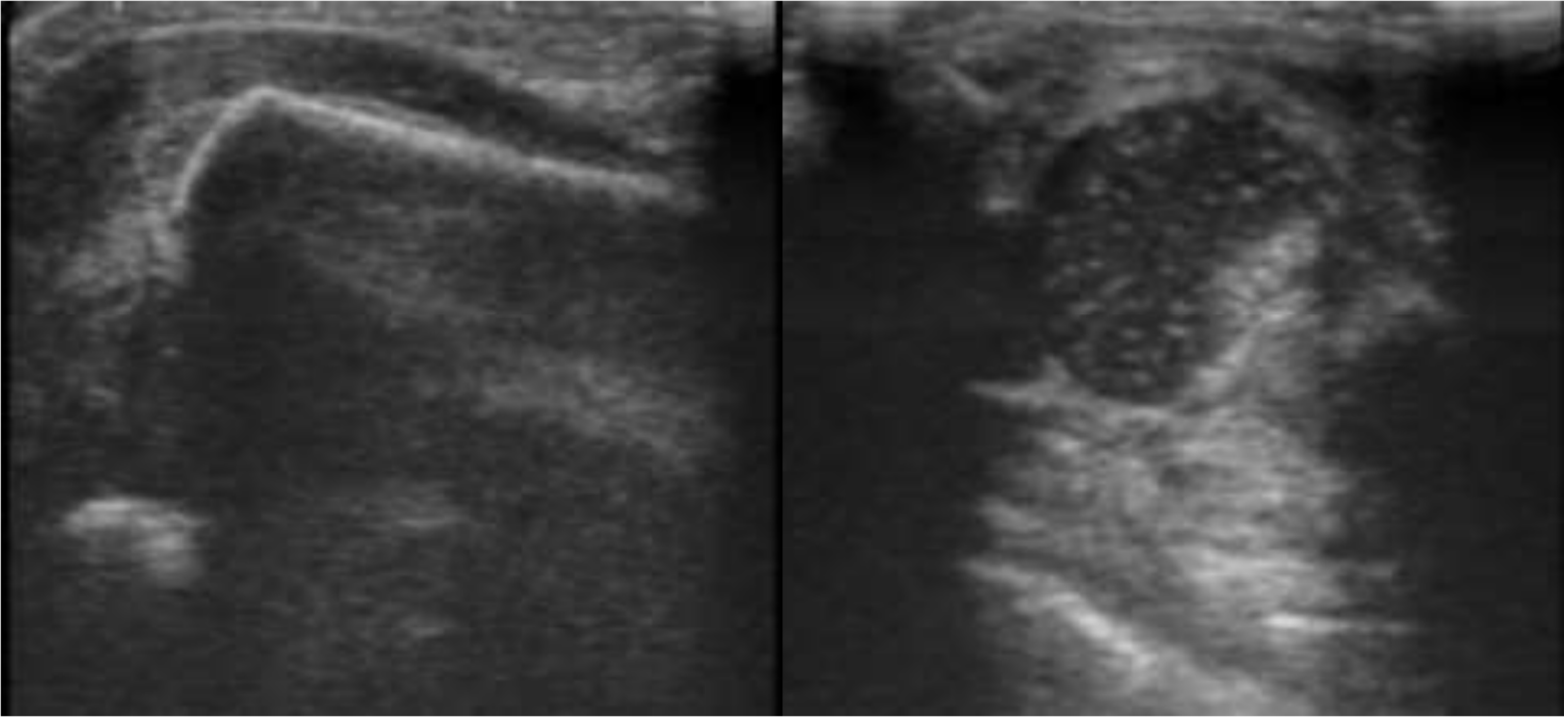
Newborn shoulder ultrasonography.
